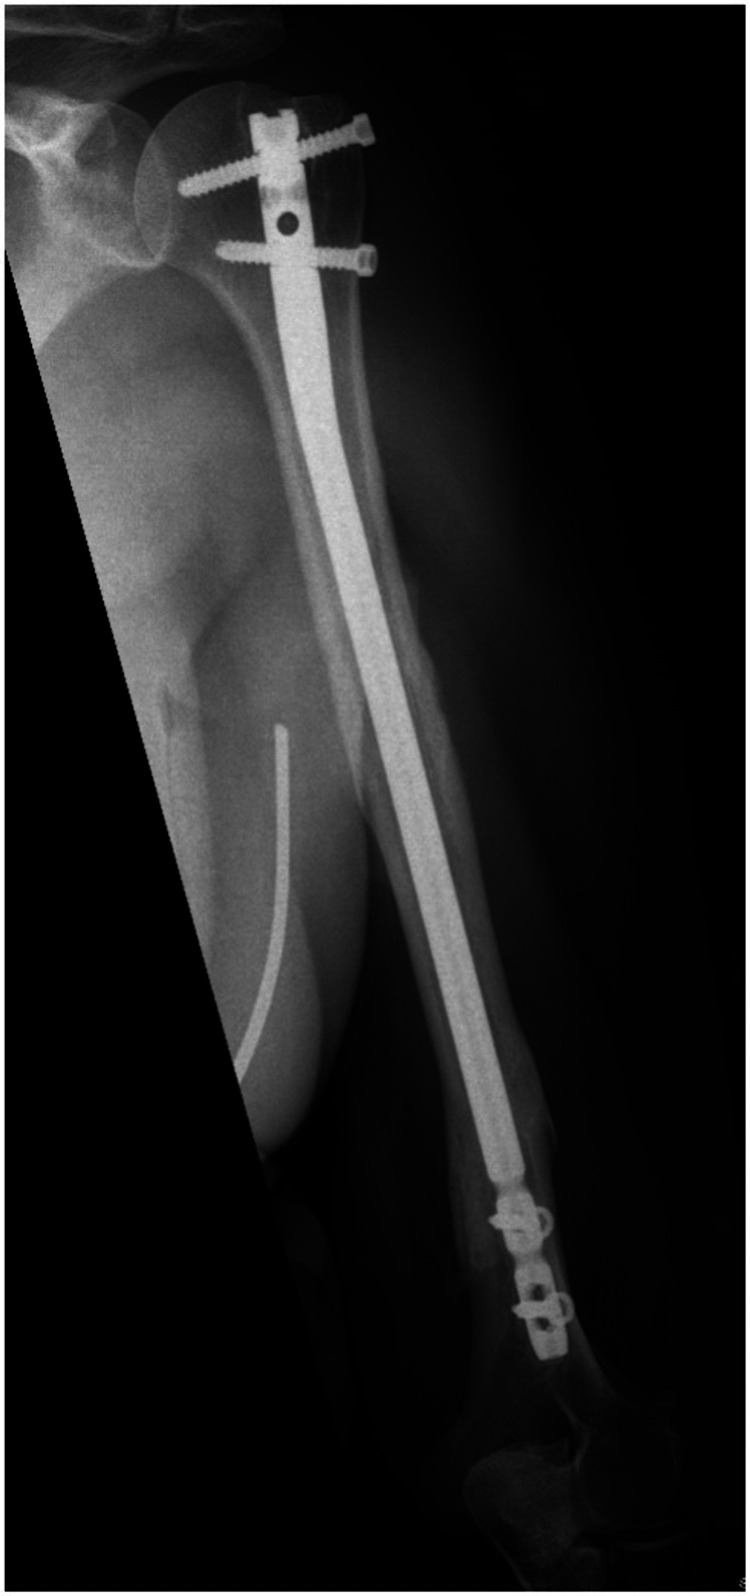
Anterior/posterior humerus radiograph demonstrating a healed segmental fracture at two year follow up.
